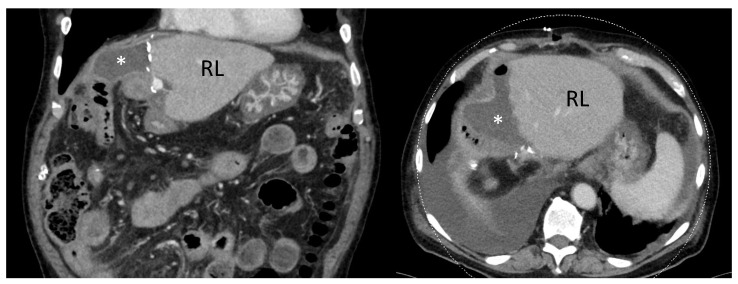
The CT scans of a patient that suffered from post-hepatectomy bilioma (white asterisk) originating from the remnant liver (RL). The left half of the image shows frontal/coronal plain, and the right half of the image shows the transverse plain.
